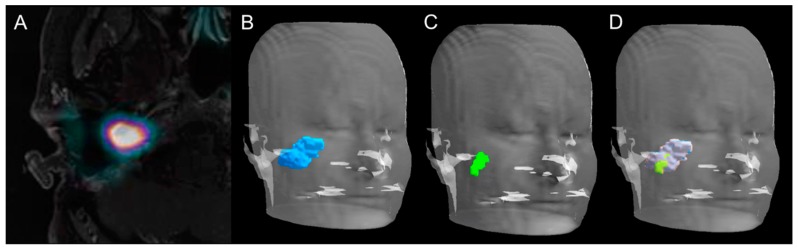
Volume rendering technique representations of GTV delineated on PET, MRI, and PET/MRI. Axial slice of combined ^18^F-FDOPA PET/MRI imaging in a 43-year-old woman (patient 5, Table 1) with a relapsing 40-mm apparently sporadic right jugulotympanic PGL previously treated with surgery (A). Volume rendering technique representation of GTV delineated on MRI (B, blue volume), ^18^F-FDOPA PET/CT (C, green volume), and combined ^18^F-FDOPA PET/MRI imaging (D, green and gray volume).
